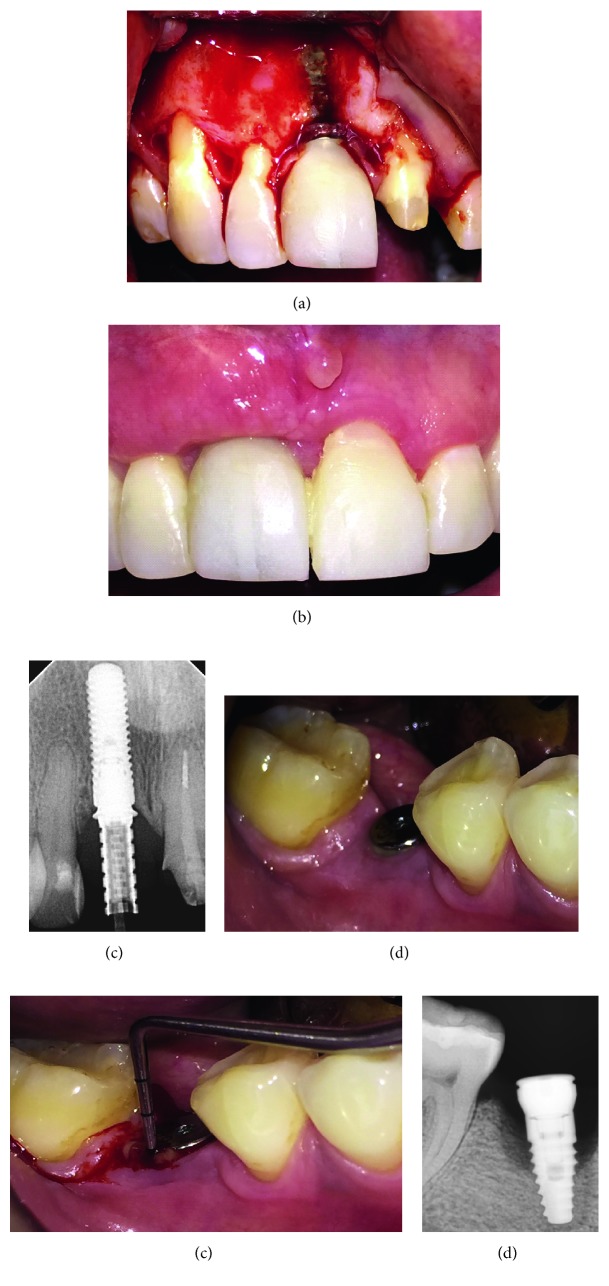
Failure cases. Patient 3: exploratory surgical procedure for peri-implantitis (a), soft tissues after a bone graft healing (b), and periapical X-ray after 4 months (c). Patient 4: intraoral photography of implant (d), periodontal probing (e), and periapical X-ray showing vertical bone loss around implant (f).
